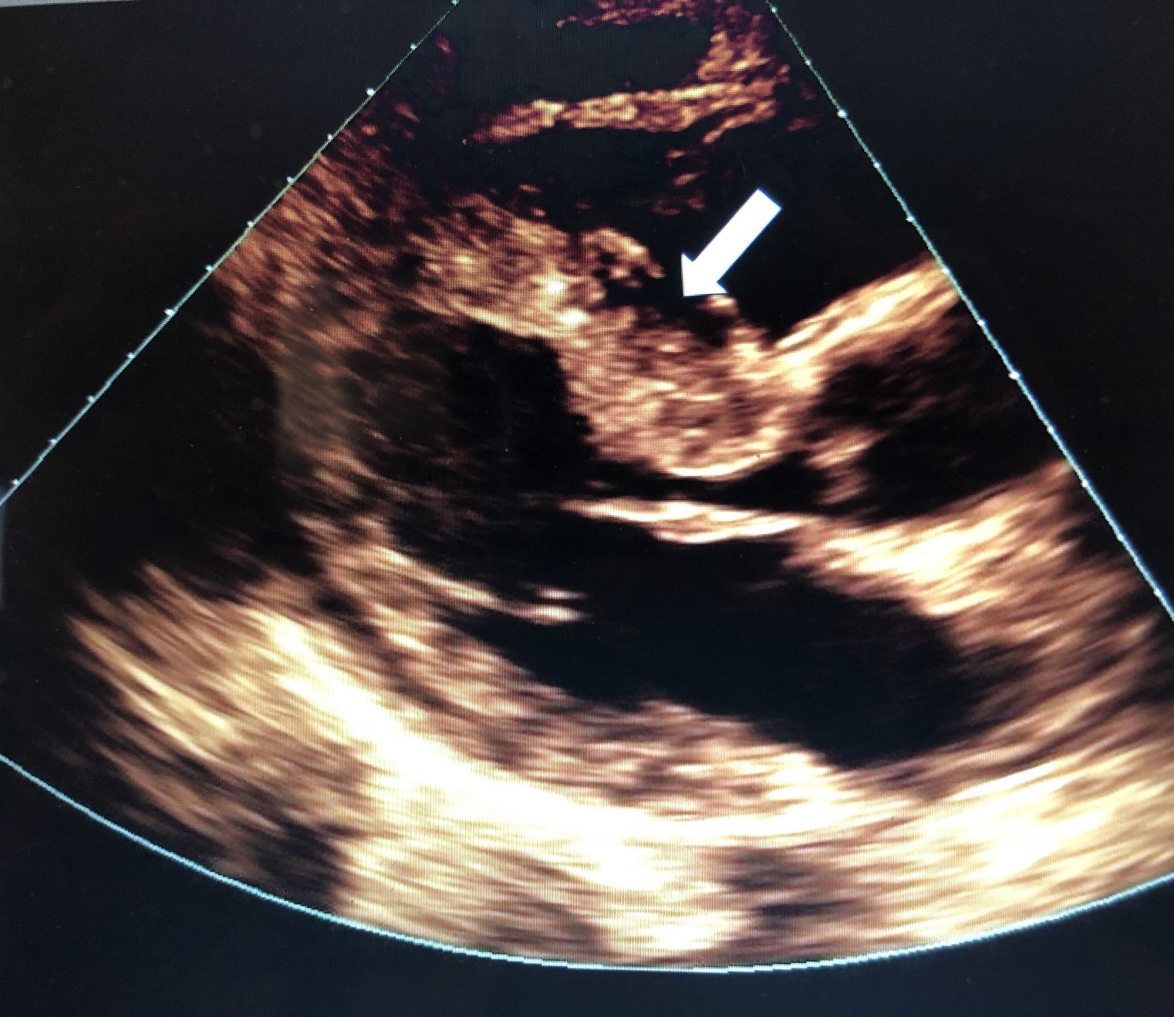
Parasternal long axis with arrow pointing to hypertrophic septum
